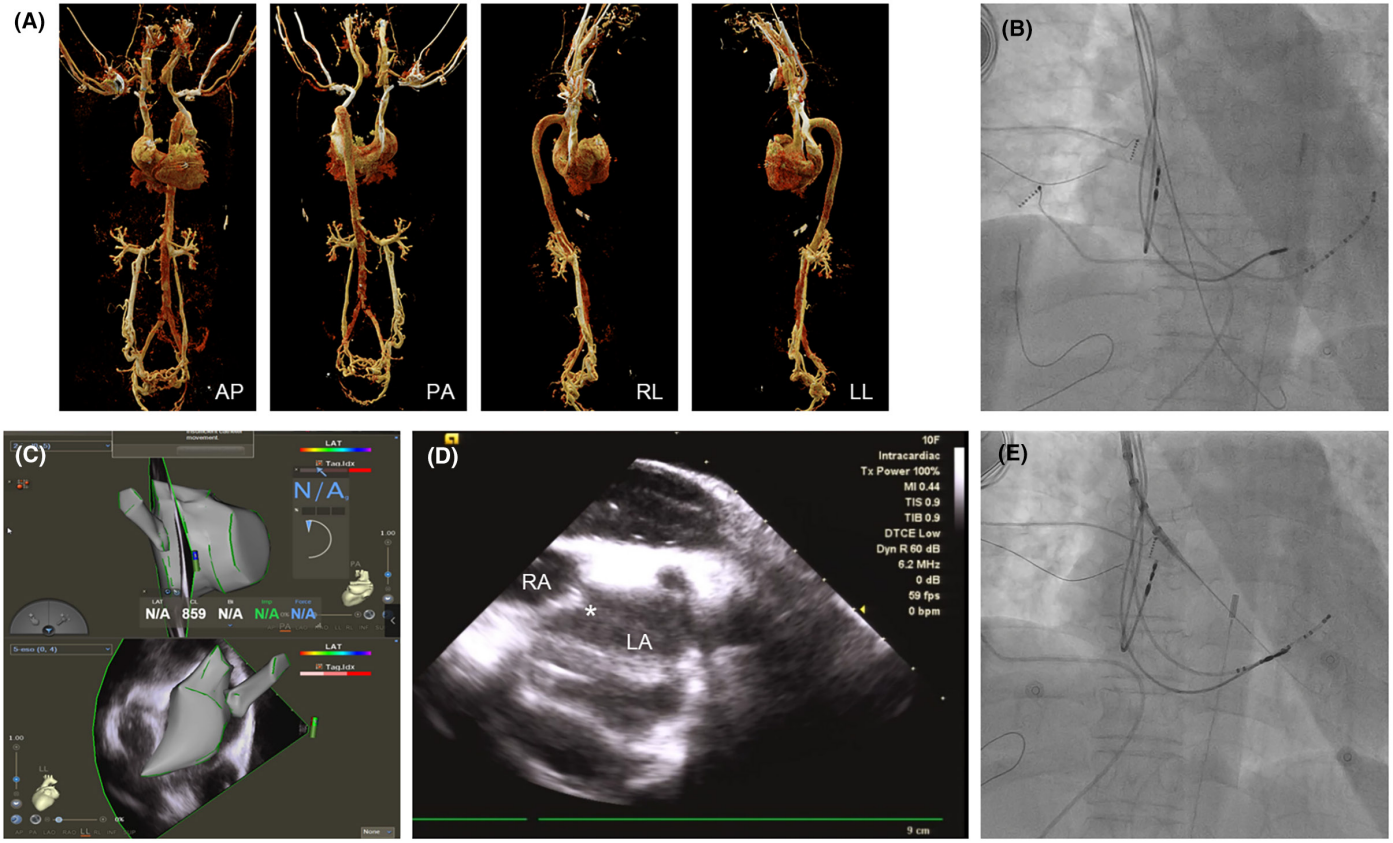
Transseptal puncture in this case. (A) CT venography of the patient with interruption of the inferior vena cava (I‐IVC) complicated with persistent left superior vena cava (PLSVC) in different projections. The patient has separated SVC and PLSVC. The azygos vein instead of IVC drained into the left subclavian vein and finally entered the right atrium through the PLSVC. (B) The decapolar catheter was placed into coronary sinus through the right jugular vein. The 10F intracardiac echocardiography (ICE) catheter was placed at the level of the LA in the azygos vein through the left femoral vein. (C) ICE modeling of the left atrium. (D) Visualization of transseptal puncture (TSP) by ICE. The asterisk indicated the sight of tenting by VIZIGO sheath. (E) The X‐ray image after TSP. The J‐tip guidewire was in the left ventricle.
